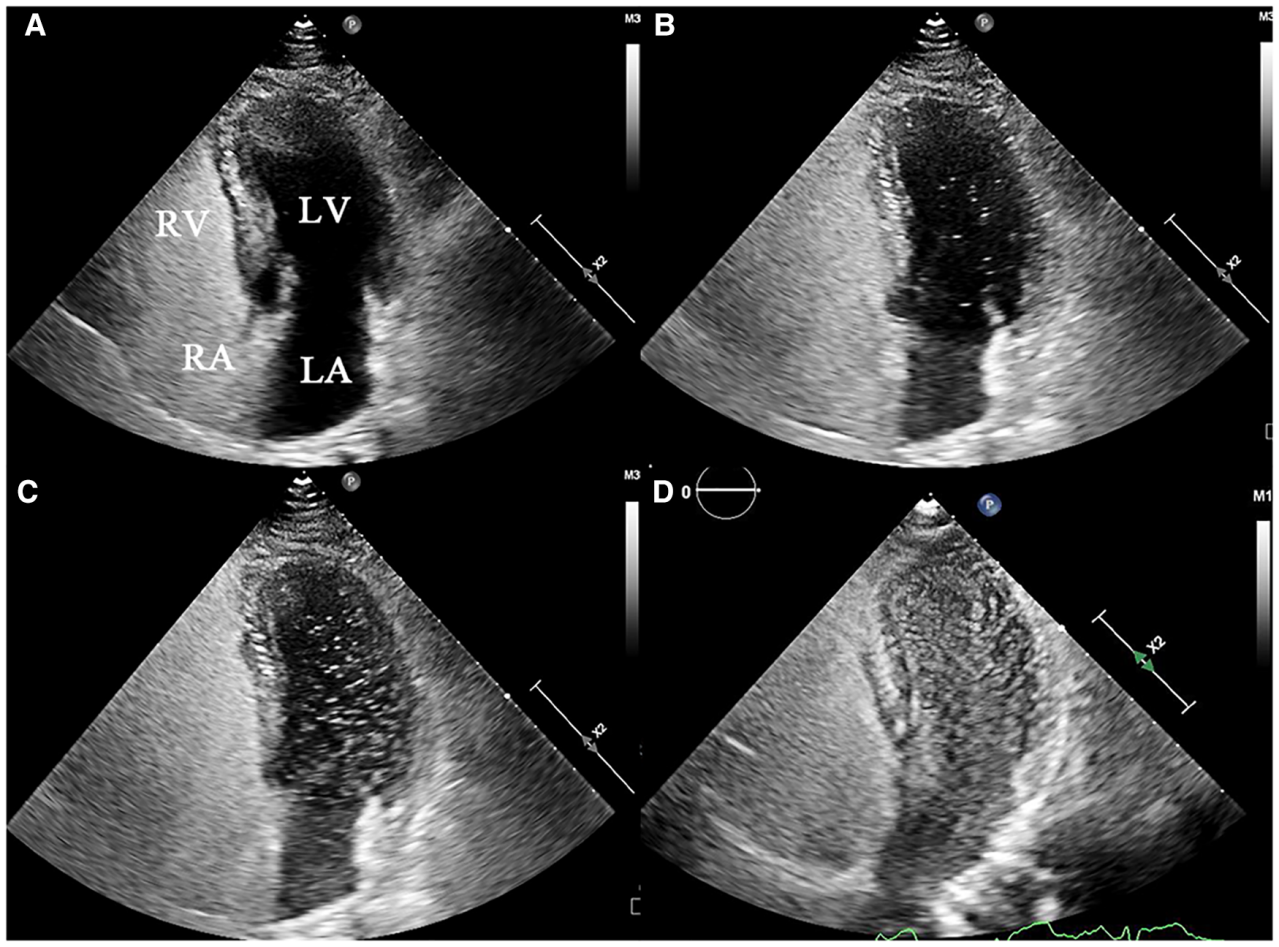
Representative echocardiograms of P-RLS grading under c-TTE (grade 0–3). (A) grade 0: negative in LV, (B) grade 1:1–30 bubbles in LV, (C) grade 2:31–100 bubbles in LV, (D) and grade 3:>100 bubbles in LV. P-RLS, pulmonary right-to-left shunt; c-TTE, contrast transthoracic echocardiography; LA, left atrium; RA, right atrium; LV, left ventricle; RV, right ventricle.
